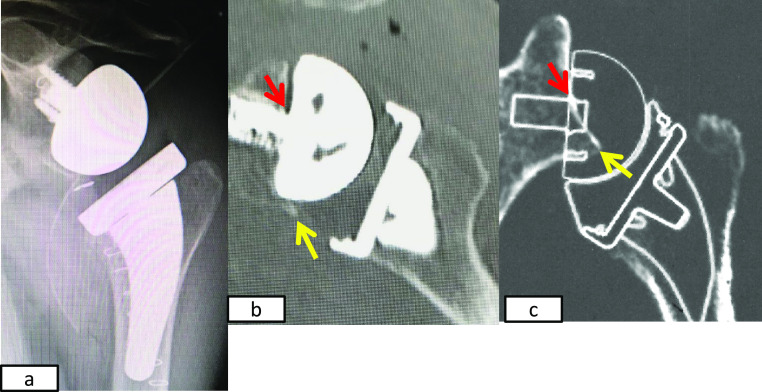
Immediately postoperative images and preoperative planning images. a) Plain radiography. b) Coronal multiplanar image. c) Preoperative planning
